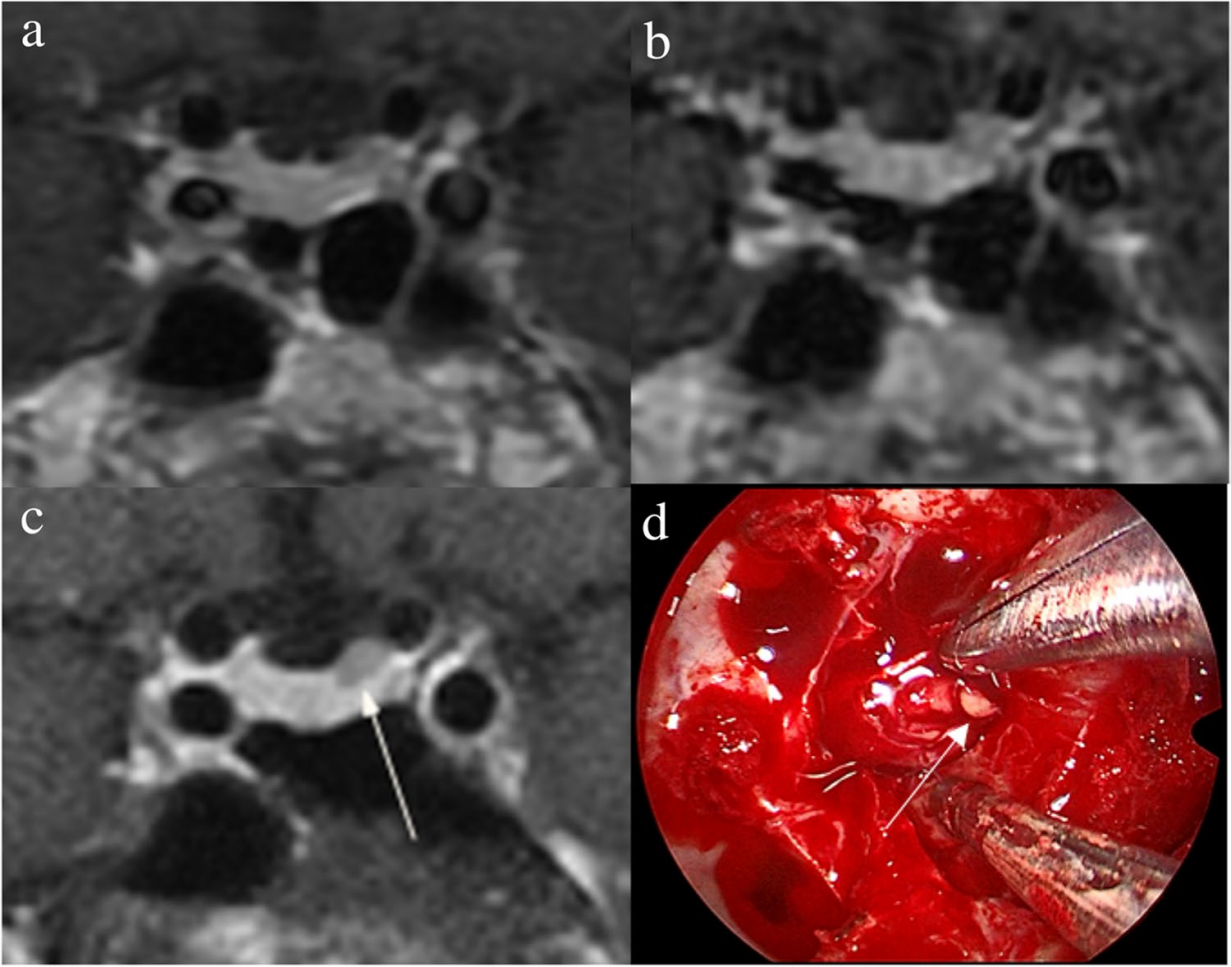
Images in a 43-year-old man with Cushing’s disease. The lesion is missed on (a) coronal contrast-enhanced T1-weighted image and (b) coronal dynamic contrast-enhanced T1-weighted image obtained with two-dimensional (2D) fast spin echo (FSE) sequence. c Coronal contrast-enhanced T1-weighted image on high-resolution MRI obtained with 3D FSE sequence shows a round pituitary microadenoma (arrow) measuring approximately 5 mm with delayed enhancement on the left side of the pituitary gland. d Intraoperative endoscopic photograph for microsurgical resection of the 5-mm pituitary microadenoma (arrow)
